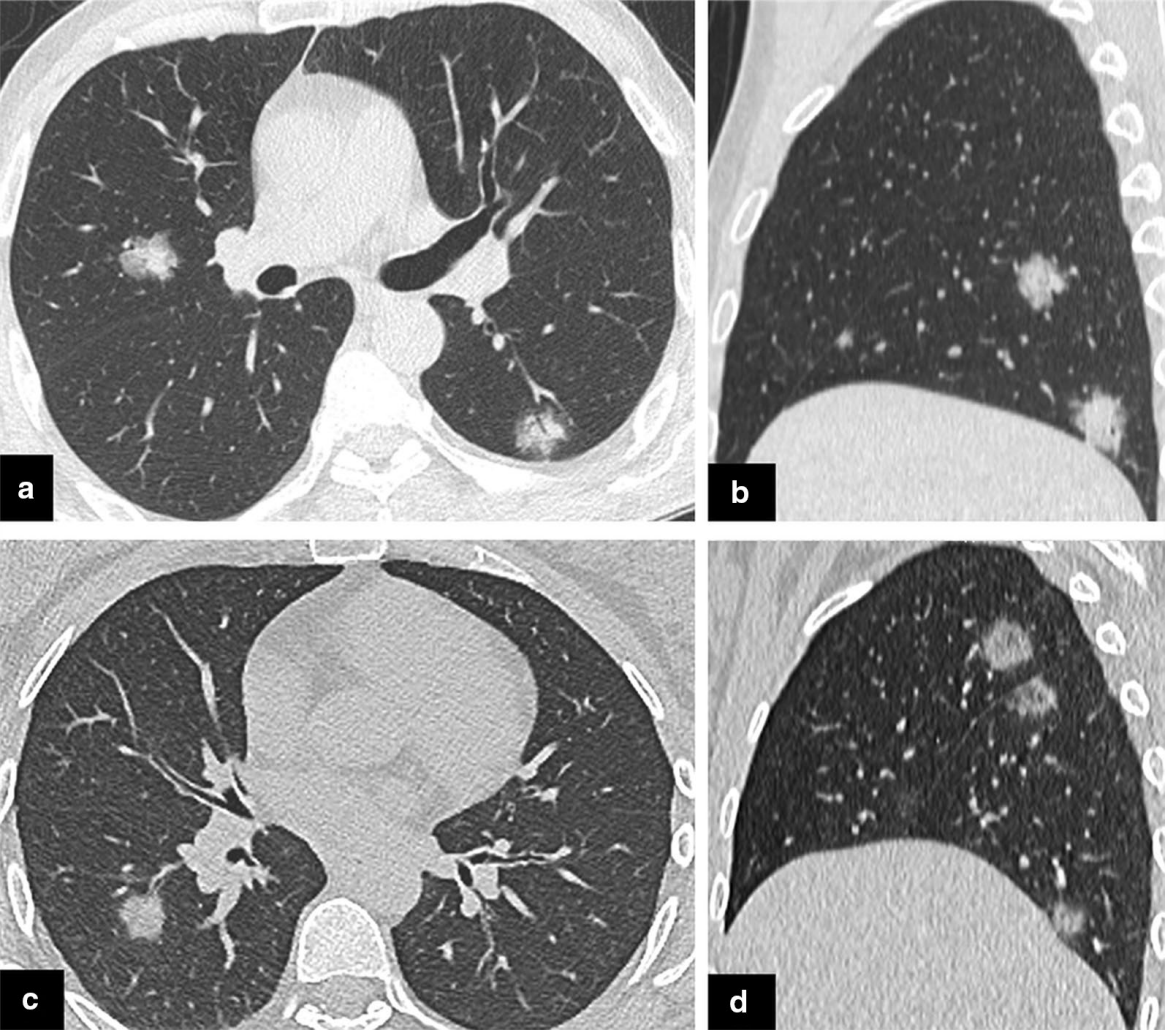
Fungal infection and COVID-19 pneumonia. a, b Axial (a) and sagittal (b) CT images show bilateral nodular opacities in a patient diagnosed with microbiologically proven aspergillosis. c, d Axial (c) and sagittal (d) CT images show nodular opacities in a COVID-19 patient. Note that the central part of the opacities in COVID-19 pneumonia has a relatively lower density (d)
